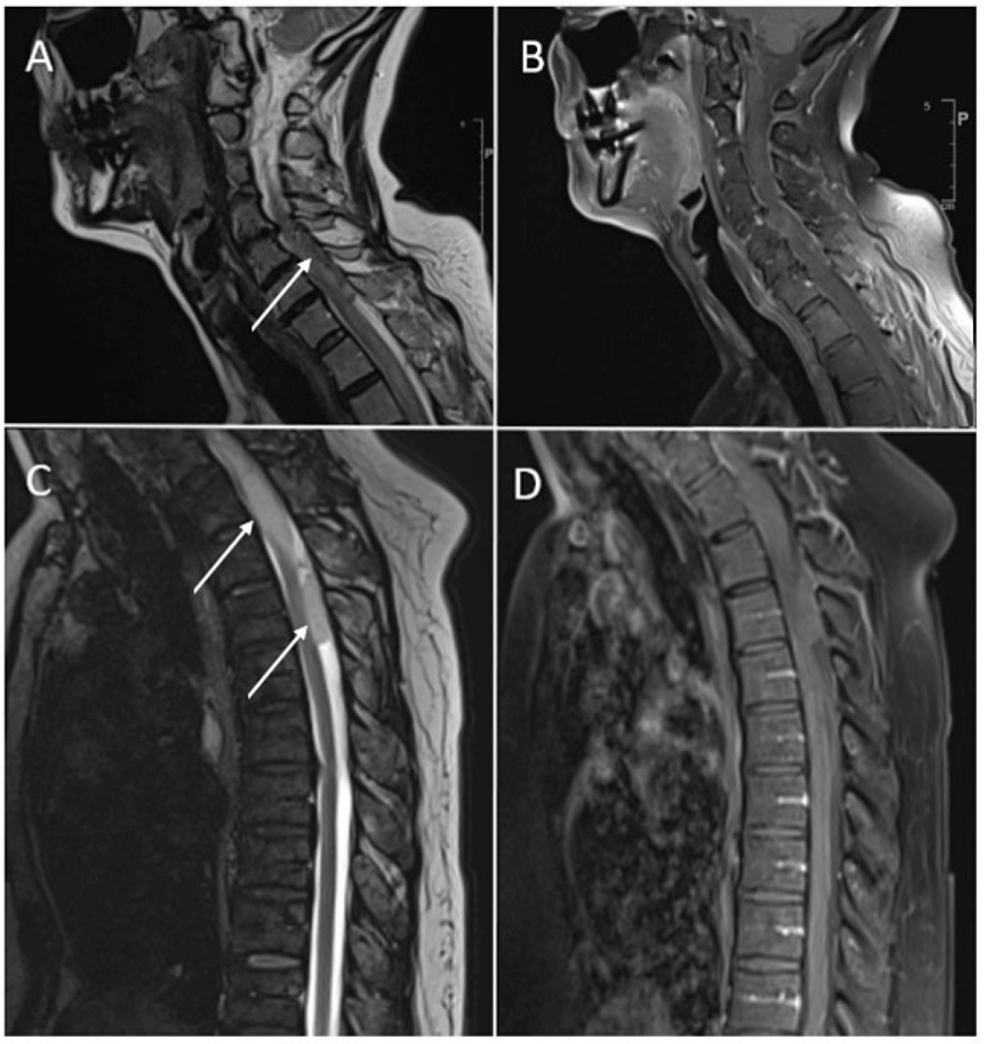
Sagittal MRI images from case one (A) T2 sequence of the patient's cervical spine showing intradural hematoma (white arrow); (B) Post-contrast T1 sequence of the patient's cervical spine showing no abnormal enhancement; (C) T2 sequence of the patient's thoracic spine showing the intradural hematoma (white arrows); (D) Post-contrast T1 sequence of the patient's thoracic spine showing no abnormal enhancement.
